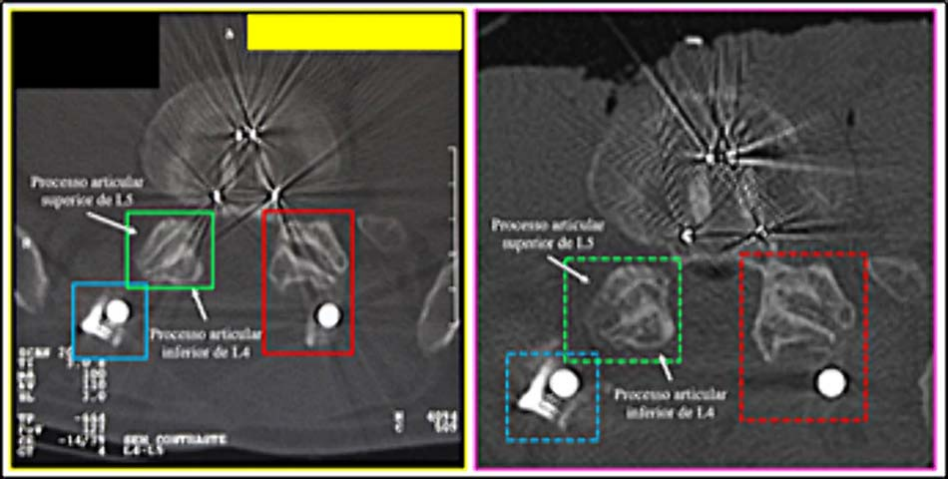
Morphology of the lower articular process of the L4 vertebra and the upper articular of the L5 vertebra. Comparison between the axial slice images of the antemortem (left) and postmortem (right) CT scans. Consistent elements.
